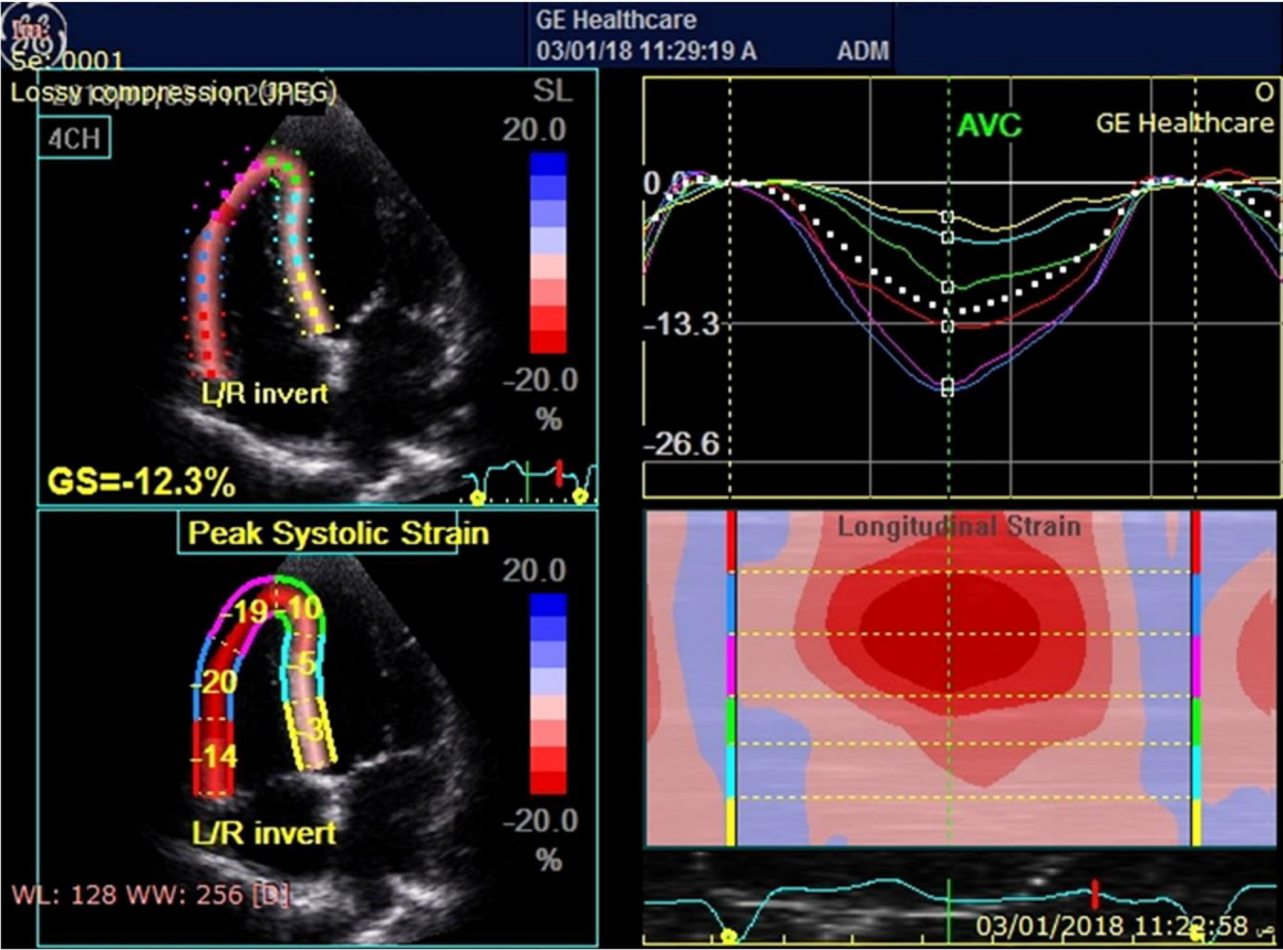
The measurement of RV GLS and RV FWLS from apical 4-chamber view. A 60-year-old male patient was considered as having normal RV function by TAPSE (2.1 cm); however, RV GLS was − 12.3% and RV FW PSS was − 17.6%
